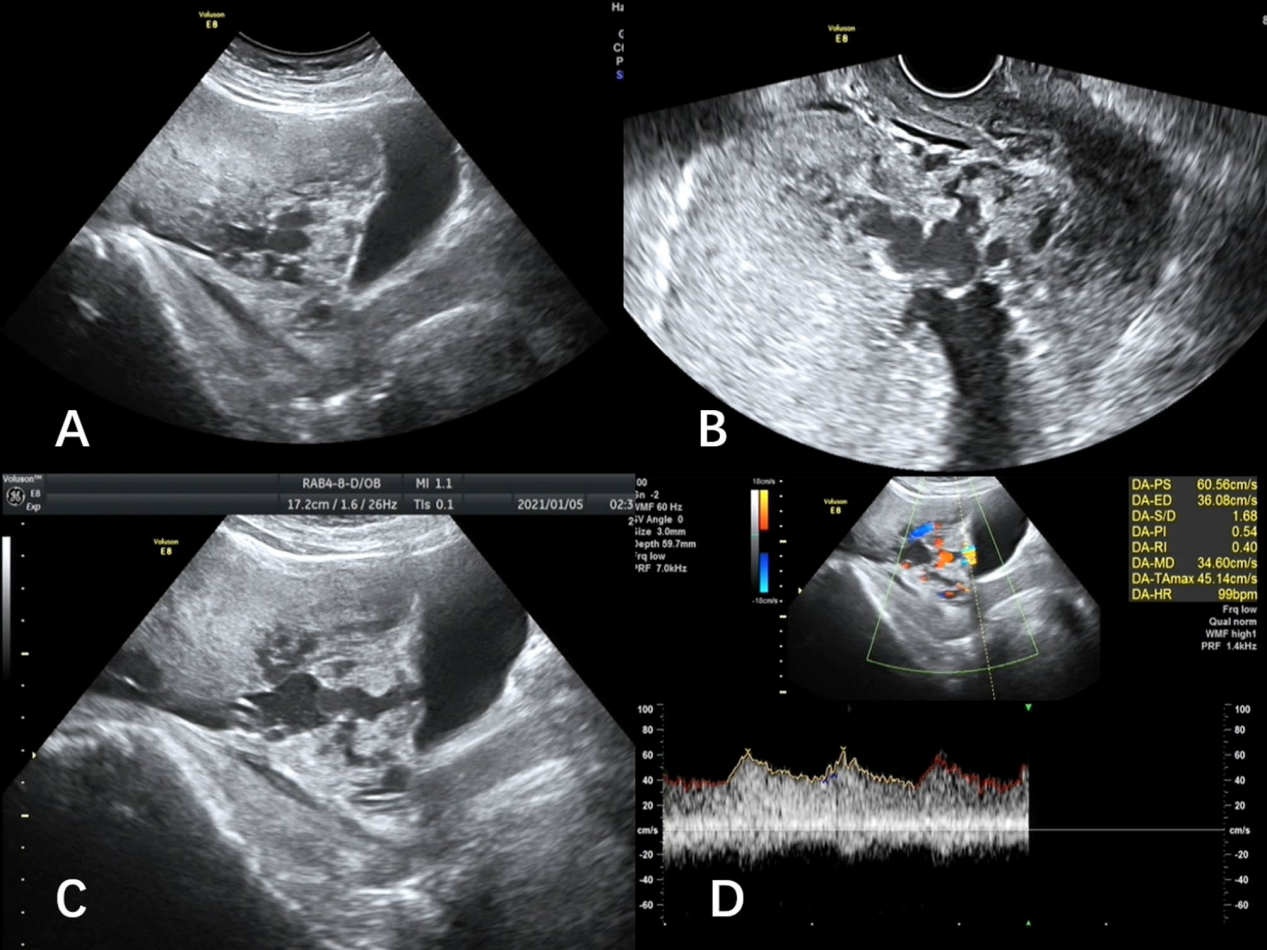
31y, G2P1. A. The woman had one previous cesarean Sect. (1 point). placenta previa (1 point), loss of the retroplacental space (1 point), and absent retroplacental myometrium (2 points). B. Transvaginal ultrasound. C. Numerous and confluent lacunae (2 points), and feeder vessels extending to the inferior anterior uterine wall. Color-doppler imaging shows numerous and confluent blood flow in the lacunae and retroplacental myometrium (2 points). The flow velocity of the arcuate artery reached 60 cm/s. D. Placental thickness is 48 mm (2 points). Total score is 11. The woman underwent cesarean section at 32 weeks of gestation. Balloon occlusion of the abdominal aorta and bilateral uterine artery embolization were performed. The placenta had penetrated the uterine wall and reached the posterior wall of the bladder. Intraoperative bleeding was 3000 ml. Bladder repair was performed
